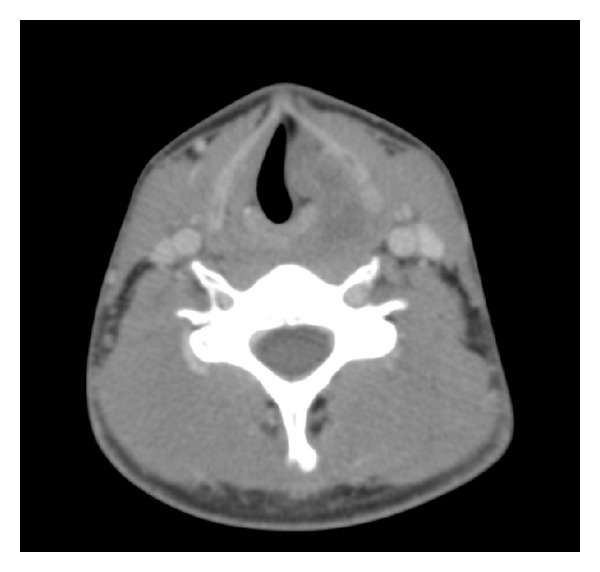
CT scan of neck, axial view, showing laryngotracheal deviation.
